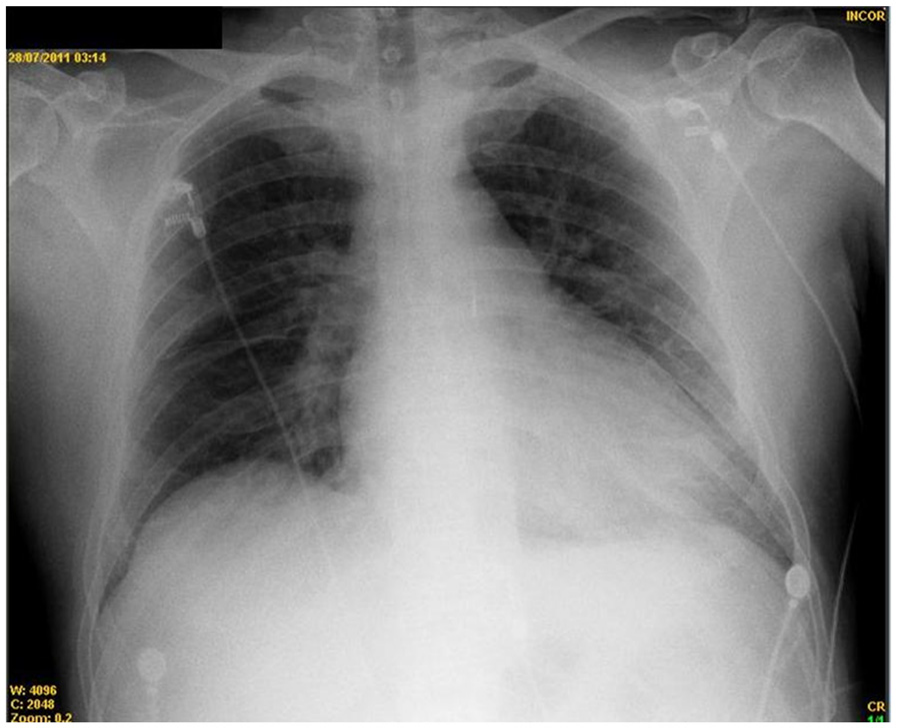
Pre-operative X-ray showing normal lung fields.
